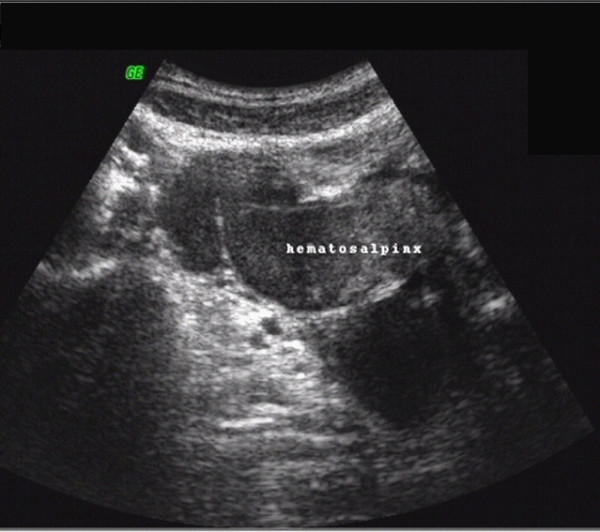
Sonographic scan revealing a right hematosalpinx. The image shows a 2 D vaginal ultrasound scan performed on the patient eight days before surgery. It reveals a hematosalpinx, measuring 45 × 33 mm, in the right Fallopian tube.
